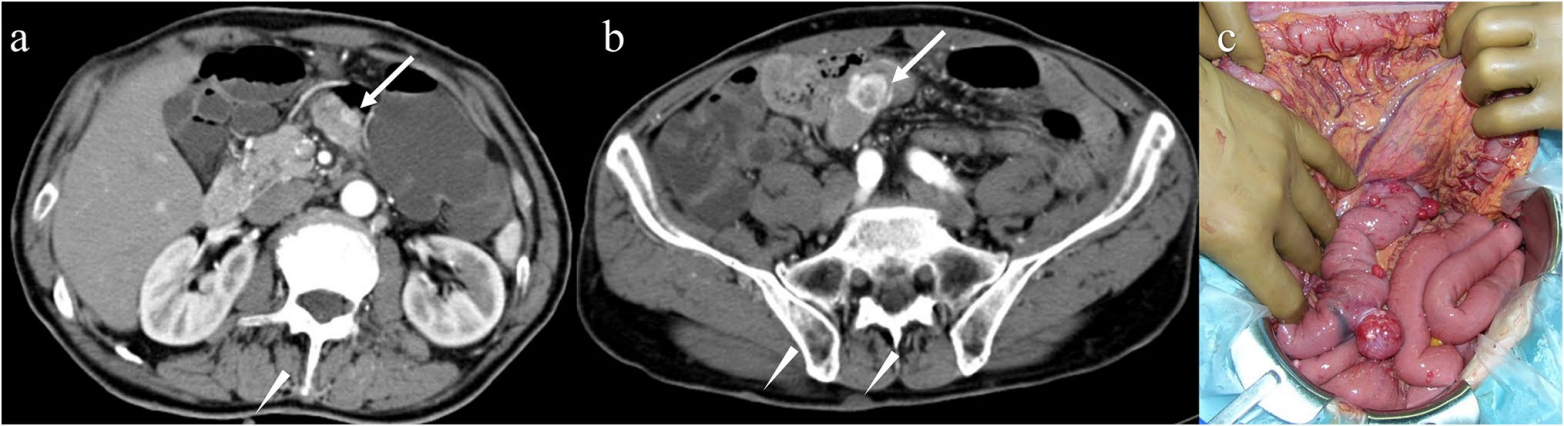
Multiple gastrointestinal stromal tumors associated with neurofibromatosis type 1. A 70-year-old male presented with melena. His hemoglobin level decreased from 14.0 g/dL to 6.0 g/dL within 1 month. He was diagnosed with neurofibromatosis type 1. Axial contrast-enhanced CT images showing multiple hypervascular tumors of various sizes in the small bowel (a, b: arrows). Note that multiple skin lesions are compatible with neurofibromas (a, b: arrowheads). Laparotomy reveals multiple exophytic nodules in the small intestine (c)
